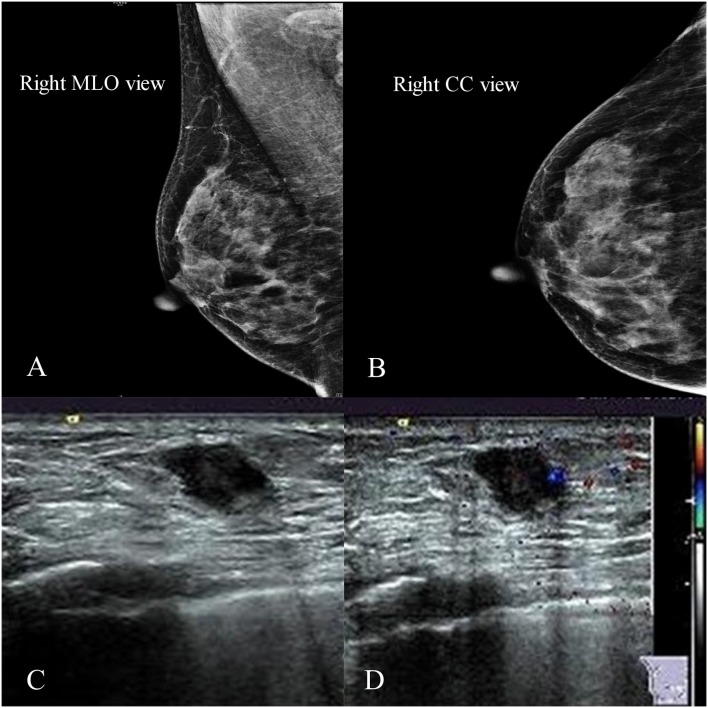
Imaging of the primary tumor. (A,B) Right mammogram showed that the local structure of the inner quadrant of the right breast was crowding. (C,D) Ultrasound showed an irregular hypoechoic mass of 1.2 × 0.8 cm located at 3–4 o'clock.
